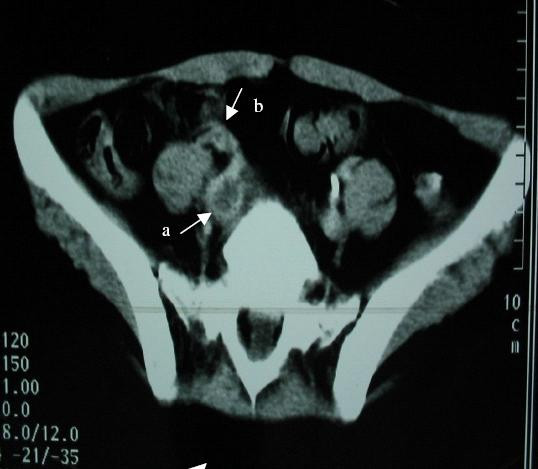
Abdominal computed tomographic scan showing an iliac vein thrombosis (a) and ascending thrombosis of the right ovarian vein (b).
